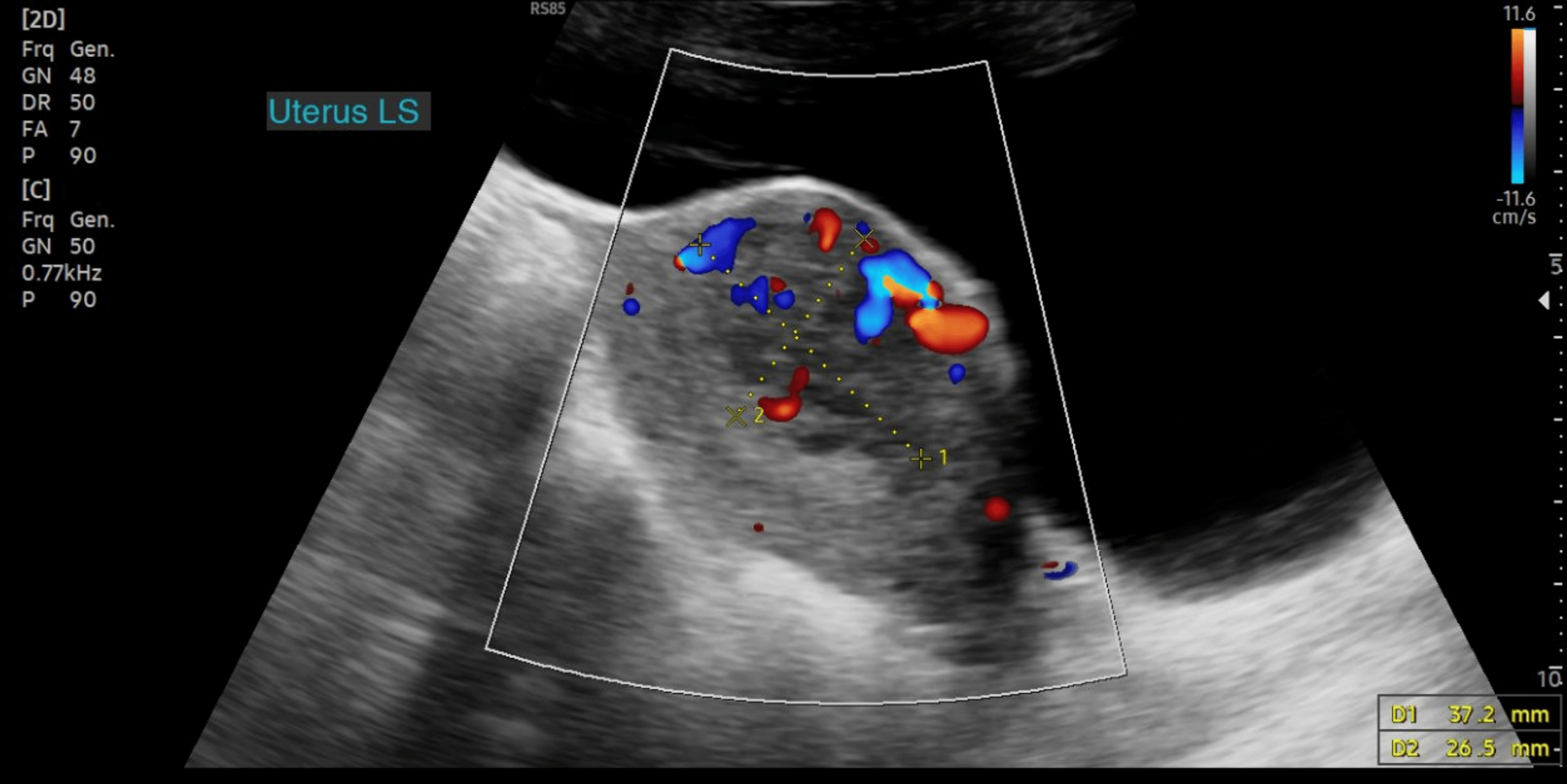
Ultrasound showing heterogenous highly vascular mass at the anterior endometrial/myometrial interface
